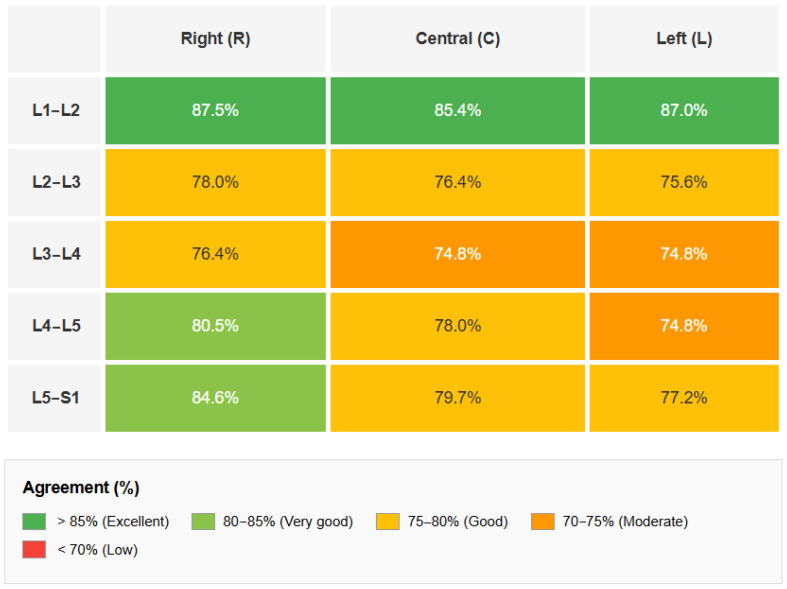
Percent agreement between dual-energy CT and Magnetic Resonance Imaging stratified by intervertebral level (L1–L2 to L5–S1) and anatomical position (right, central, left).
